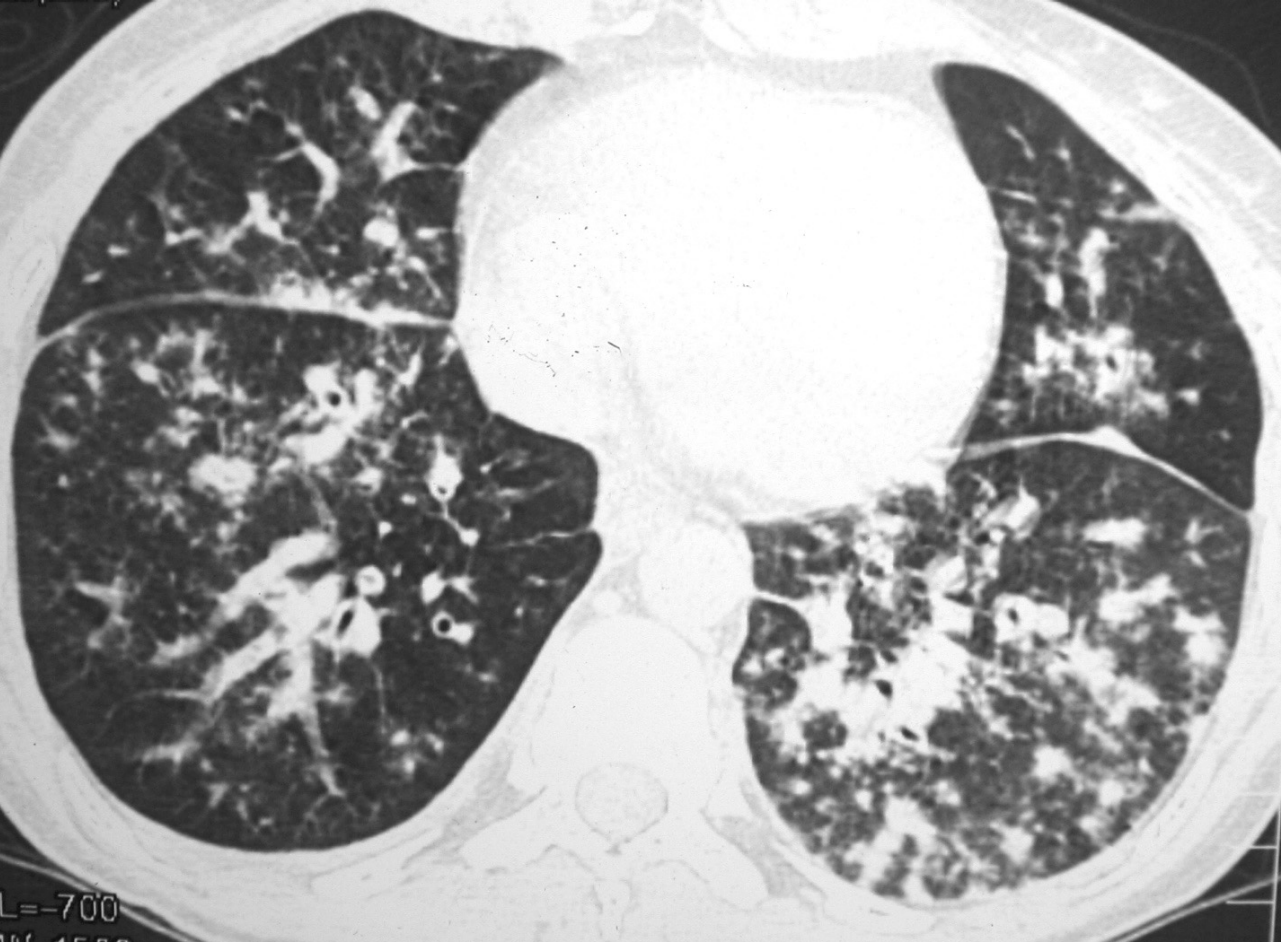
HRCT scan obtained with lung window shows diffuse peribronchovascular thickening and small nodules bilaterally. There are also nodules in the fissures and interlobular septal thickening.
